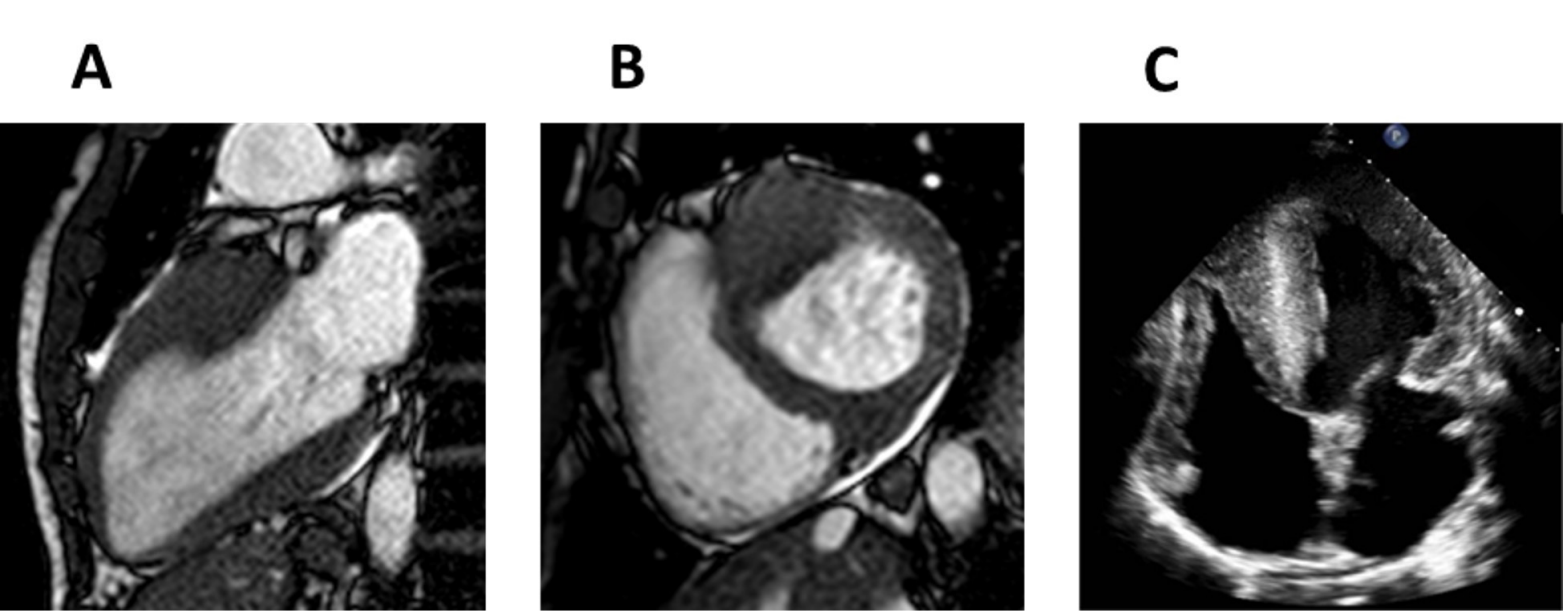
Asymmetrical septal hypertrophy mimicking hypertrophic cardiomyopathy in a patient with Fabry disease. Long-axis (A) and short-axis (B) CMR images and an echocardiography apical four-chamber view (C) of a patient with Fabry disease showing an asymmetrical septal hypertrophy that mimics the pattern seen in hypertrophic cardiomyopathy.
